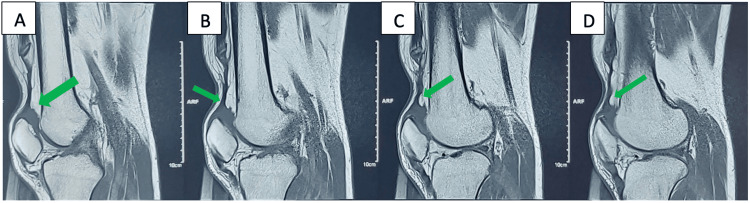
Magnetic resonance imaging (MRI) of the left knee. (A, B, C, D) Sagittal views demonstrating a complete rupture of the quadriceps tendon near its superior patellar insertion, resulting in a significant proximal tendon retraction of 5 cm. The tendon rupture and its proximal retraction, along with the avulsed bone fragment from the superior pole of the patella (clearly identified in panels C and D), are indicated by the green arrows.
